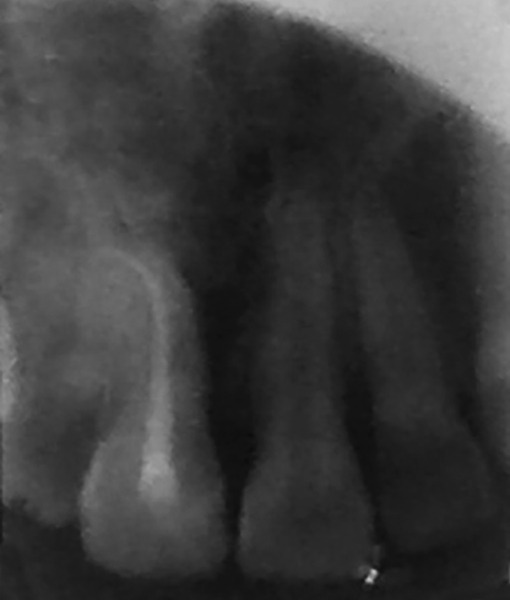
IOPA radiograph showing slight resorption on mesial surface
